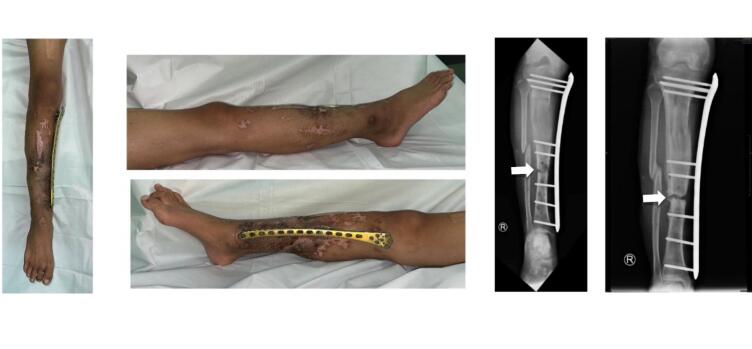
Images of the fracture and external fixation using locking compression plate. Radiograph images of the leg post LCP application (right).
